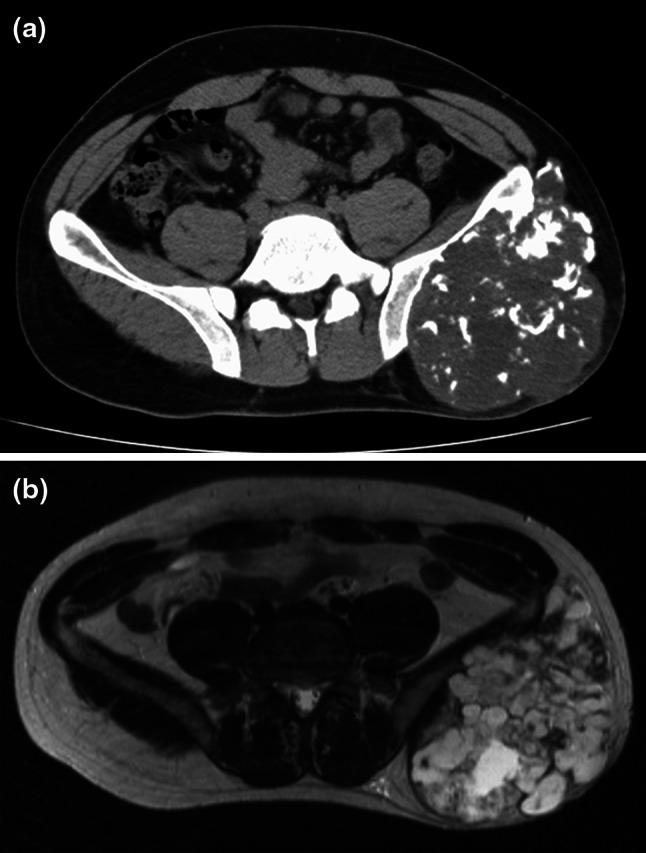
Axial CT shows an expansile tumor that originates from the posterior aspect of the ilium and contains foci of calcification (a). T2-weighted axial MR images demonstrate a lobulated lesion of the left ilium with heterogeneous high signal intensity, consistent with chondroid matrix (b)
